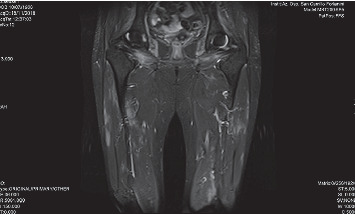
Evidence of diffuse and symmetric muscles oedema of all the muscles of the thigh at MRI.
